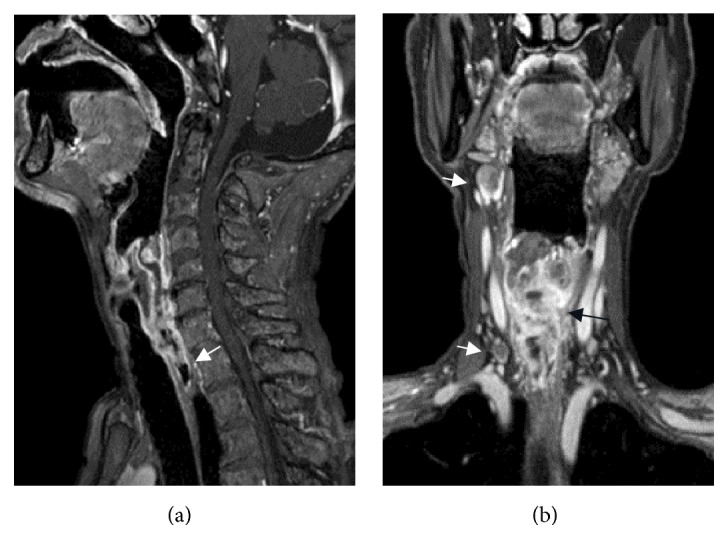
Preoperative cross-sectional MRI of the neck. (a) An enhanced sagittal image shows the tumor extending to the cervical esophagus (arrow). (b) An enhanced coronal image shows the large tumor completely occupying the hypopharyngeal space (black arrow). Note multiple lymph node metastases in the right neck (white arrows).
